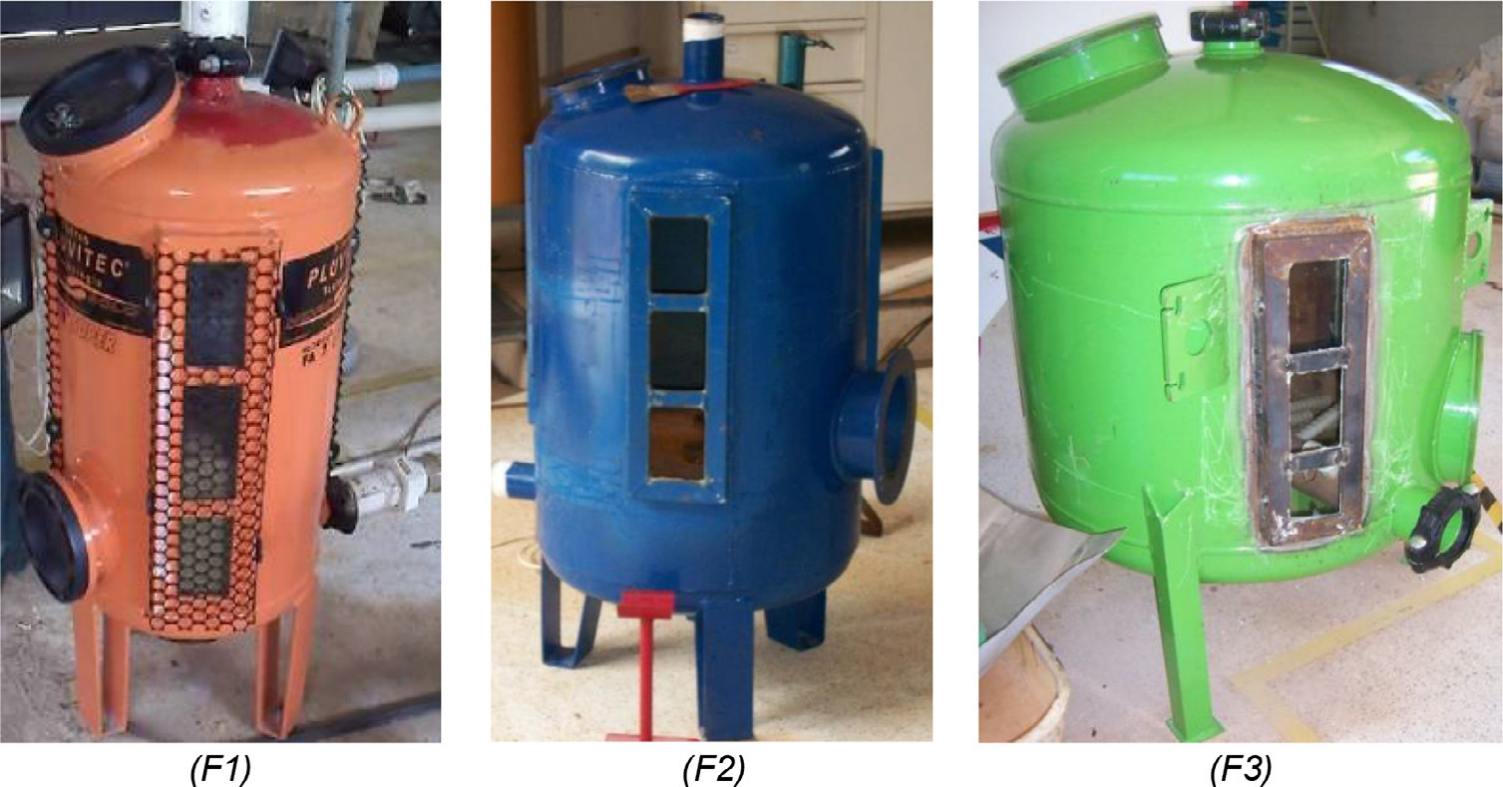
Commercial sand filters models evaluated, with detail of the tempered glass side panels installed.For the structural characterization of sand filters, it is recommended that the following technical specifications are informed by the manufacturers: Diameter of the filter; Filtration surface; Internal diameter of the inlet pipe; Internal diameter of the outlet pipe; Useful height of the filter (Distance between the base of the diffuser plate and the upper level of the drains); Number of drains; Average width of a drain opening; Number of openings per drain; Opening area per drain unit; Total opening area of the drainage system; and Ratio between drainage area and filter area.-Needle valves: the purpose of using this type of valve was to submit greater precision to the evaluations for the effective maintenance of the flow in time. Two valves must be used, one before and one after the evaluated sand filter.-Flow rate sensor: to record the flow information over time it was used an eletromagnetic flow rate sensor (Signet 2551, of the Georg Fischer brand, Piping Systems Ltd., São Paulo, Brazil), and to instantly measure the flow it was used an ultrasonic flow rate sensor (UFM170, FMS, São Paulo, Brazil). The location of the sensors followed recommendations of the standard ASABE (1993) [8]. The standard establishes that the flow sensors must have a minimum precision of ± 2% in the evaluated flow range, and must be installed respecting a minimum length of 50 times the pipe internal diameter after the valve in the flow direction, and a minimum of five times the pipe internal diameter after the flow rate sensor. Table 1 shows the technical specifications of the flow rate sensors used.
